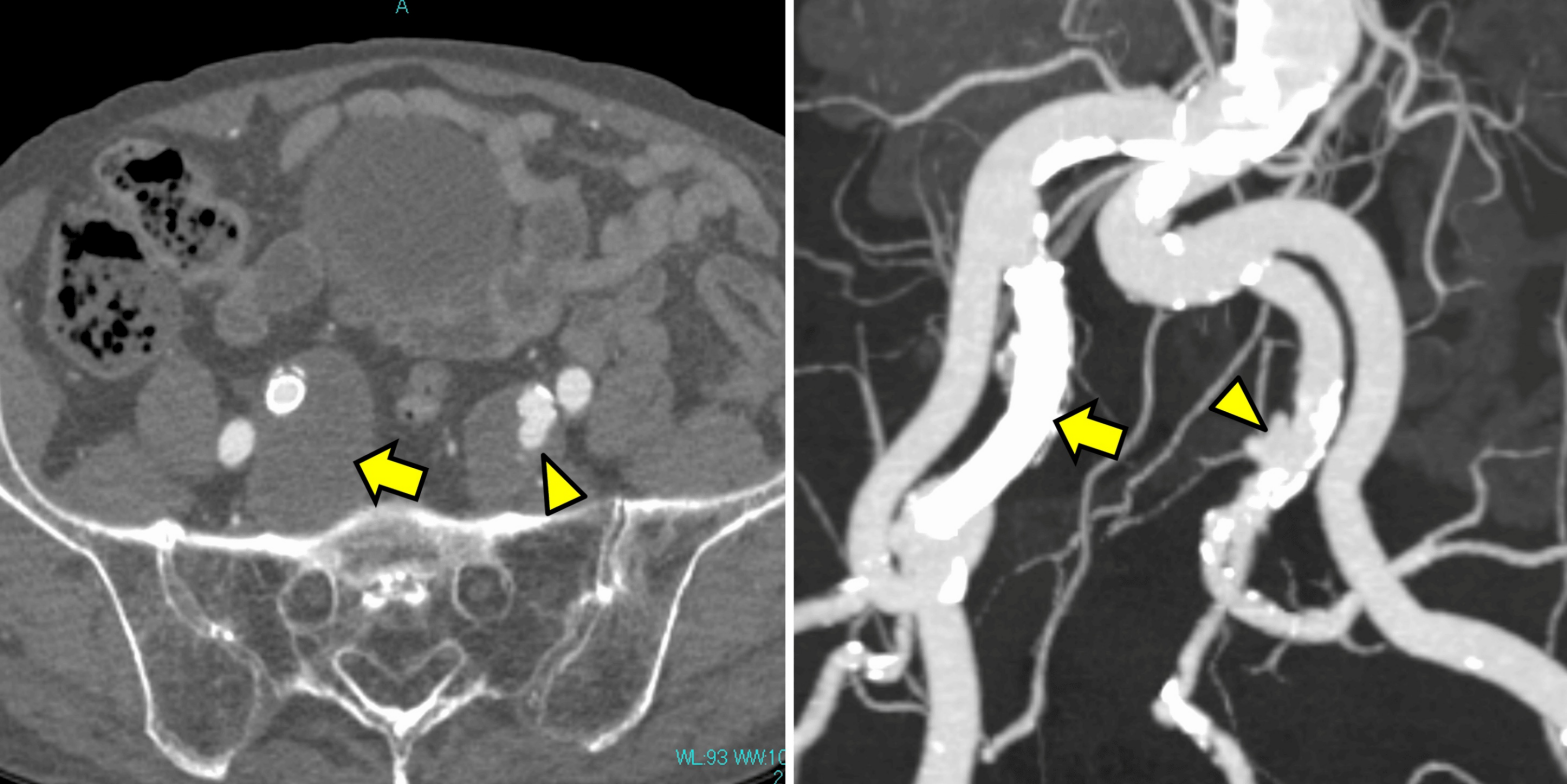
Contrast-enhanced CT five months after endovascular therapy. (Left) The small cranial false lumen of the left internal iliac aneurysm remained, similar to the previous scan (yellow arrowhead), whereas the large caudal false lumen was spontaneously thrombosed and occluded. (Right) The stent placed in the right internal iliac artery remains patent, and the false lumen of the aneurysm is completely thrombosed and occluded (yellow arrow).
